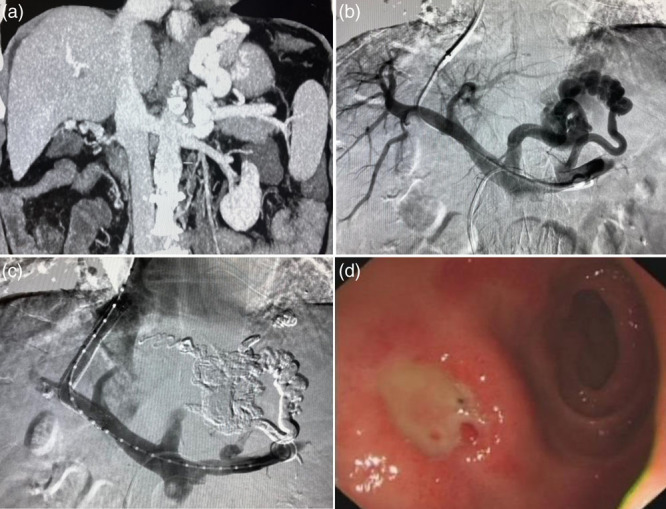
A 67-year-old male bleeding from IGV1. (a) Before the BAATO + TIPS procedure, enhanced CT showed solitary GVs bolus and GRS. (b) During the procedure, the portography showed GVs feeded of posterior gastric vein and short gastric vein; (c) post-stenting venogram showed that cyanoacrylate spreaded and embolized GVs all over. (d) After the BAATO + TIPS procedure, endoscopic examination showed the complete regression of GVs. CT, computed tomography; GRS, gastrorenal shunt.
